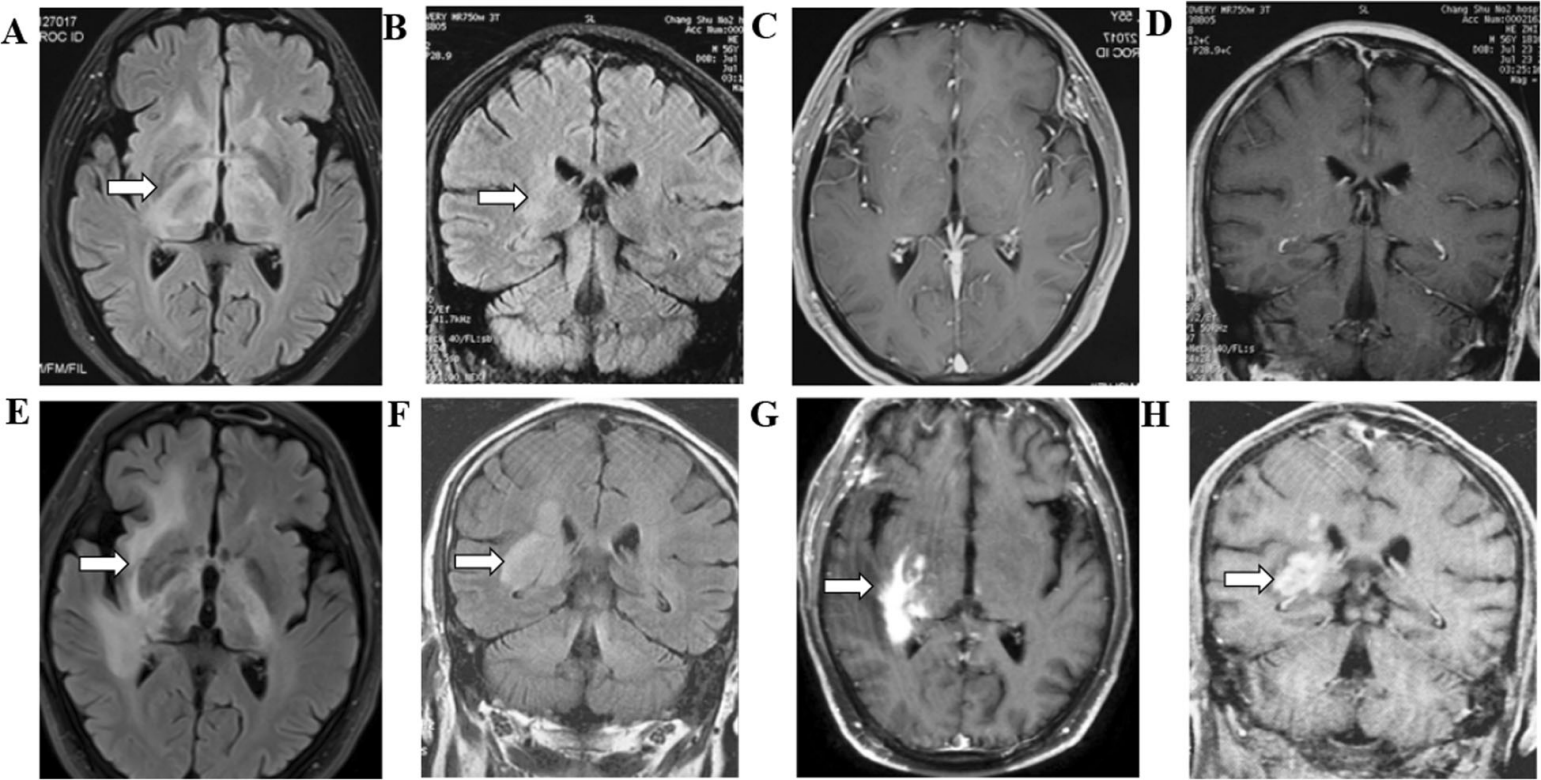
SCNSL without enhancement. Multiple lesions in the brain parenchyma showed hyperintensity on T2 Flair images (a and b; arrows). No enhancement was observed on MRI (c and d). Two months later, with the progression of the disease, the volume of lesions was observed on T2 Flair (e and f: arrows), strong enhancement was present after gadolinium injection in T1-weighted images (g and h: arrows), and stereotactic biopsy confirmed DLBCL CNS involvement
